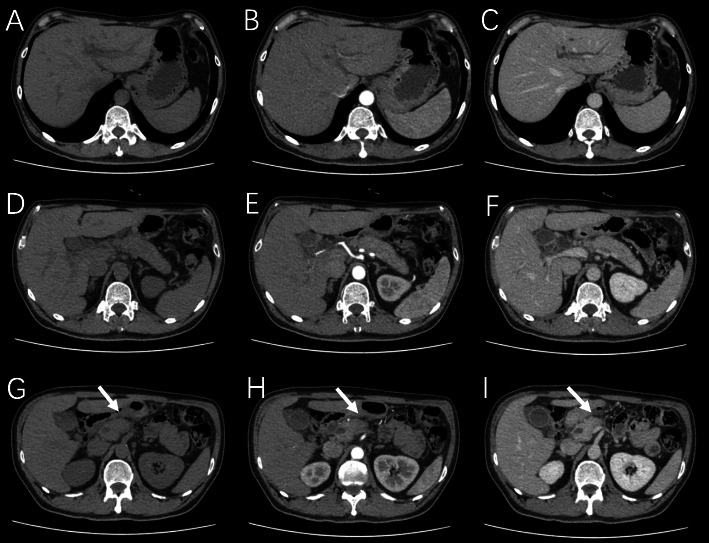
Thin-slice enhanced CT scan of pancreas showed the uncinate process of pancreatic head lesion, suggesting possible malignancy with intrahepatic and extrahepatic bile duct dilatation
